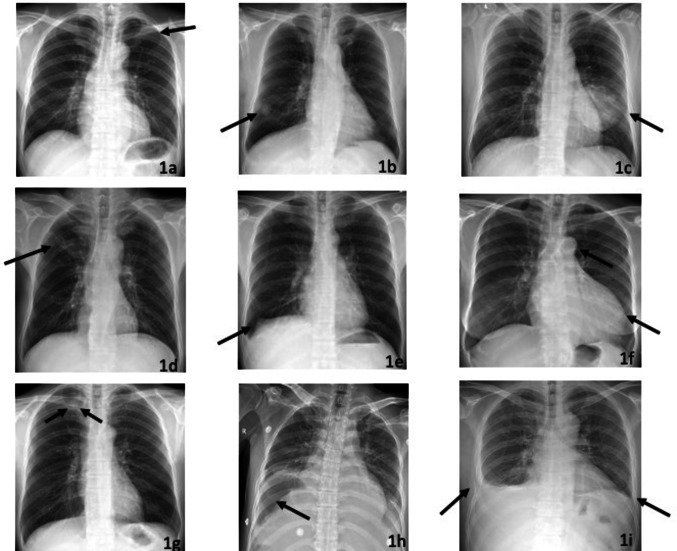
Typical radiographs showing chest abnormalities. a A small amount of pneumothorax on the left side. b A lung nodule in the right lower lobe. c A lung mass in the left lower lobe. d A cord shadow in the right upper lung lobe. e Thickening of the right pleura. f Aortic calcification and an enlarged heart shadow. g A cavity shadow in the right upper lung lobe. h Free air under the right diaphragm. i Fracture of the 7th rib on the right, with pleural effusion on both sides
